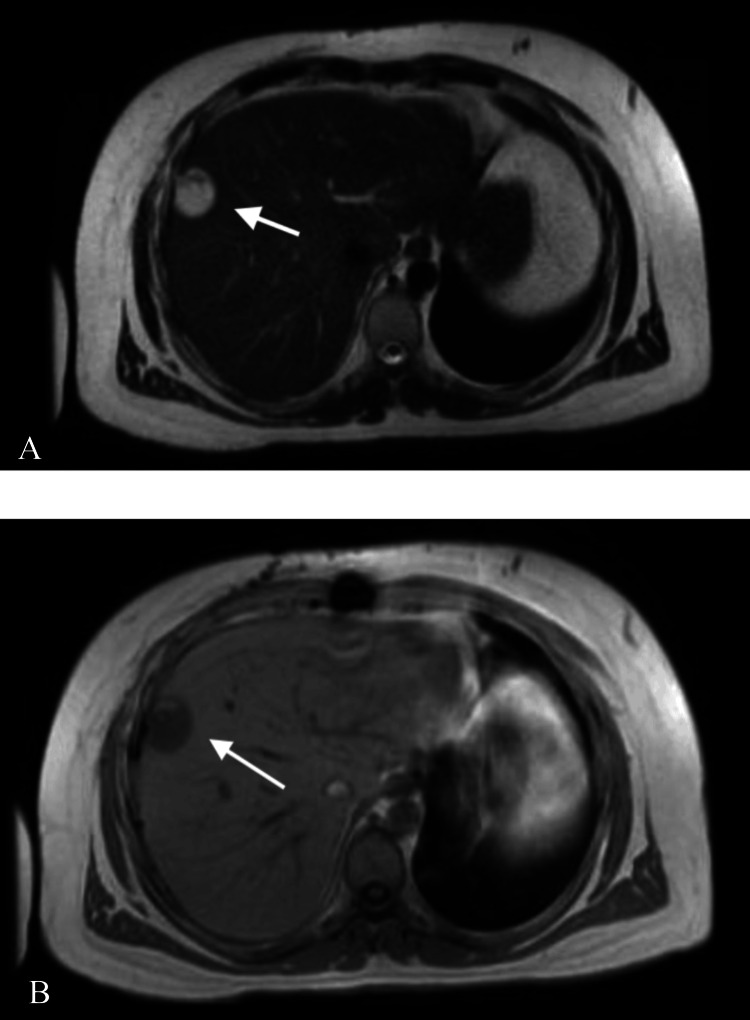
Magnetic resonance imaging of the liver. Axial MRI imaging of the abdomen [(A) T1 sequence and (B) T2 sequence] showing peripherally located liver mass.
